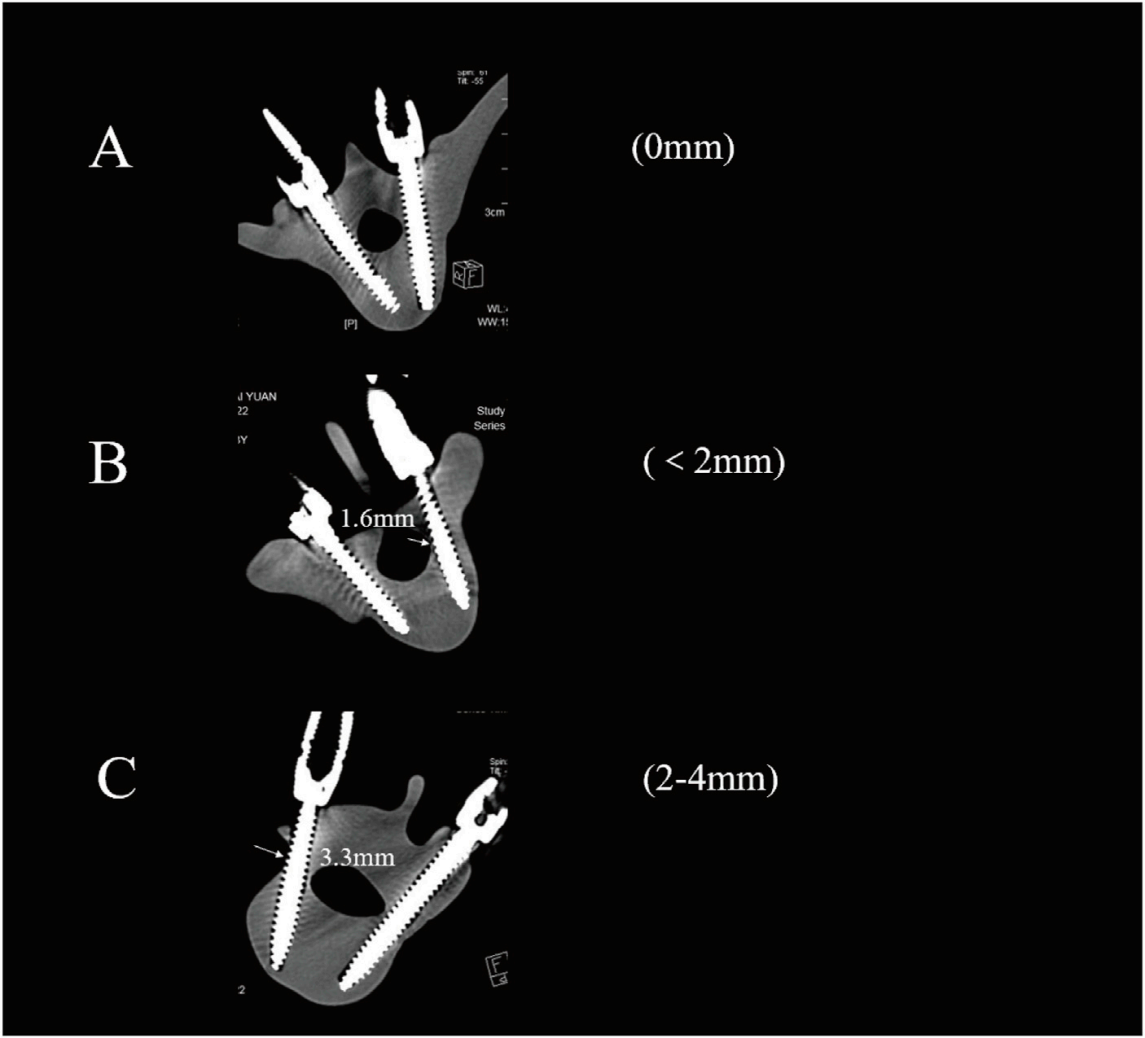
Axial CT scans classify pedicle screws (based on Gertzbein - Robbins). Grade (A) screws are fully within the pedicle (0 mm offset); Grade (B) have < 2 mm cortical penetration; Grade (C) have 2–4 mm penetration.
